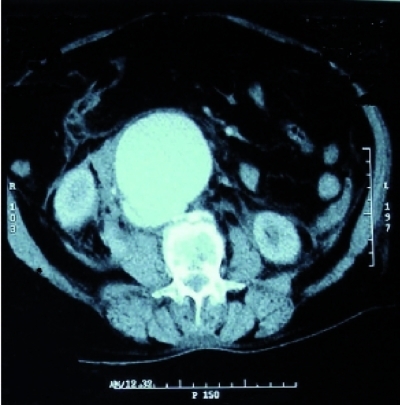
Abdominal CT scan showing infrarenal aortic aneurysm
